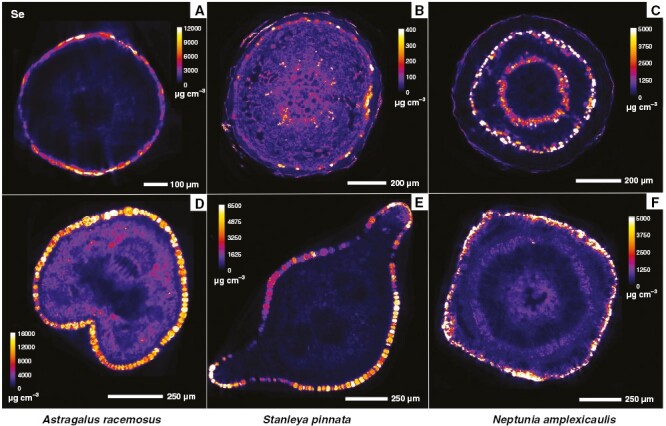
Synchrotron µXRF-CT elemental maps showing the distribution of Se in fresh hydrated roots (A–C) and petioles (D–F) in reconstructed virtual cross-sections of *Astragalus racemosus* (A, D), *Stanleya pinnata* (B, E) and *Neptunia amplexicaulis* (C, F).
